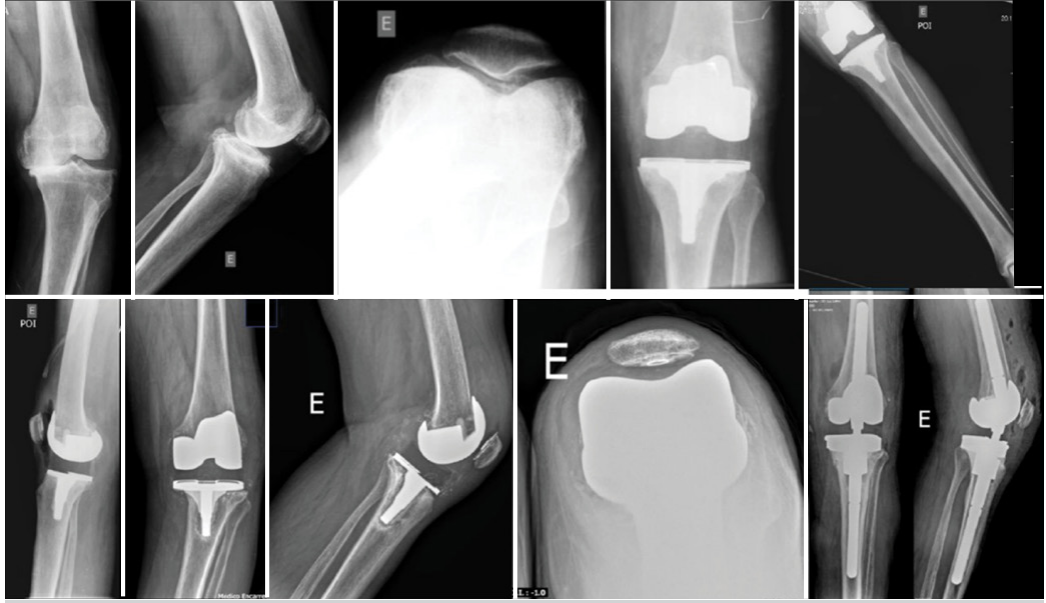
The X-ray shows the failure of the implant with constricted polyethylene, evolving with revision with a hinge-type implant.
